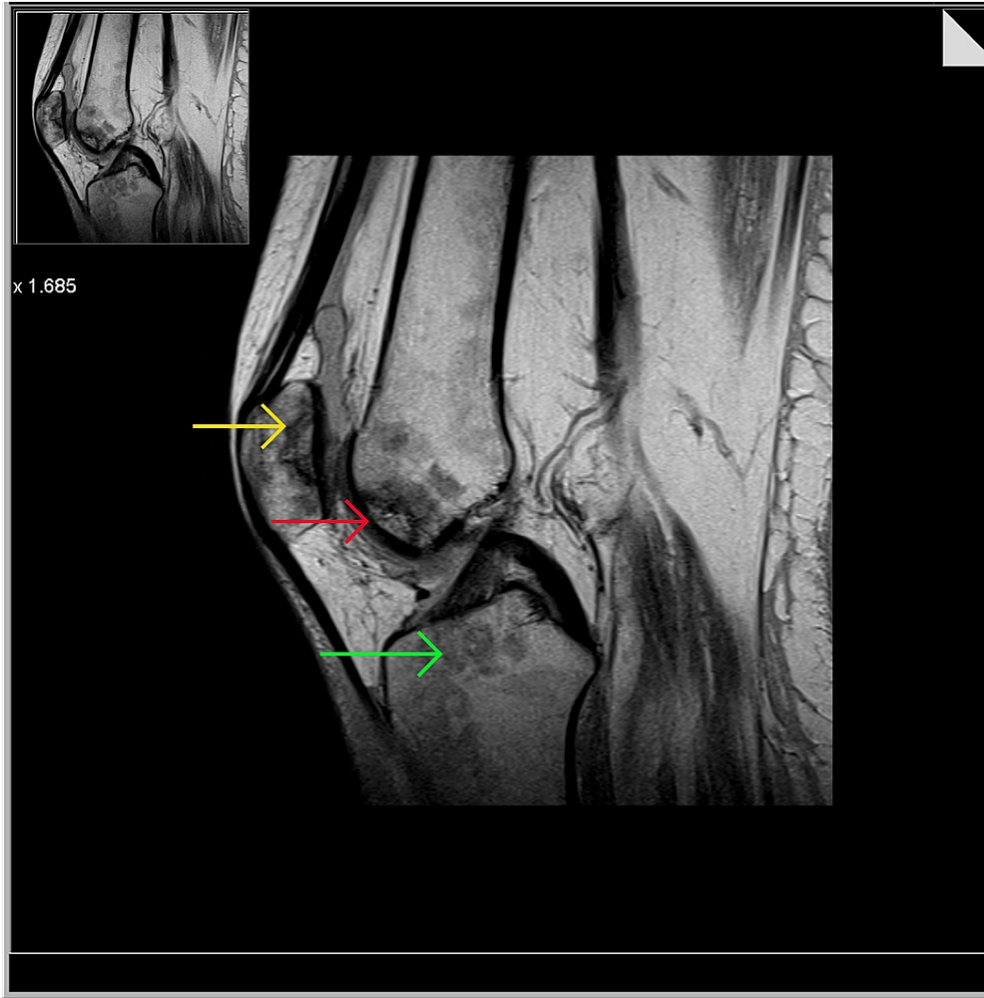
Knee MRI sagittal view showing avascular necrosis of the patella Yellow arrow is showing serpingous lines in the patella; red arrow is showing serpingous lines; green arrow is showing bone marrow heterogeneity
